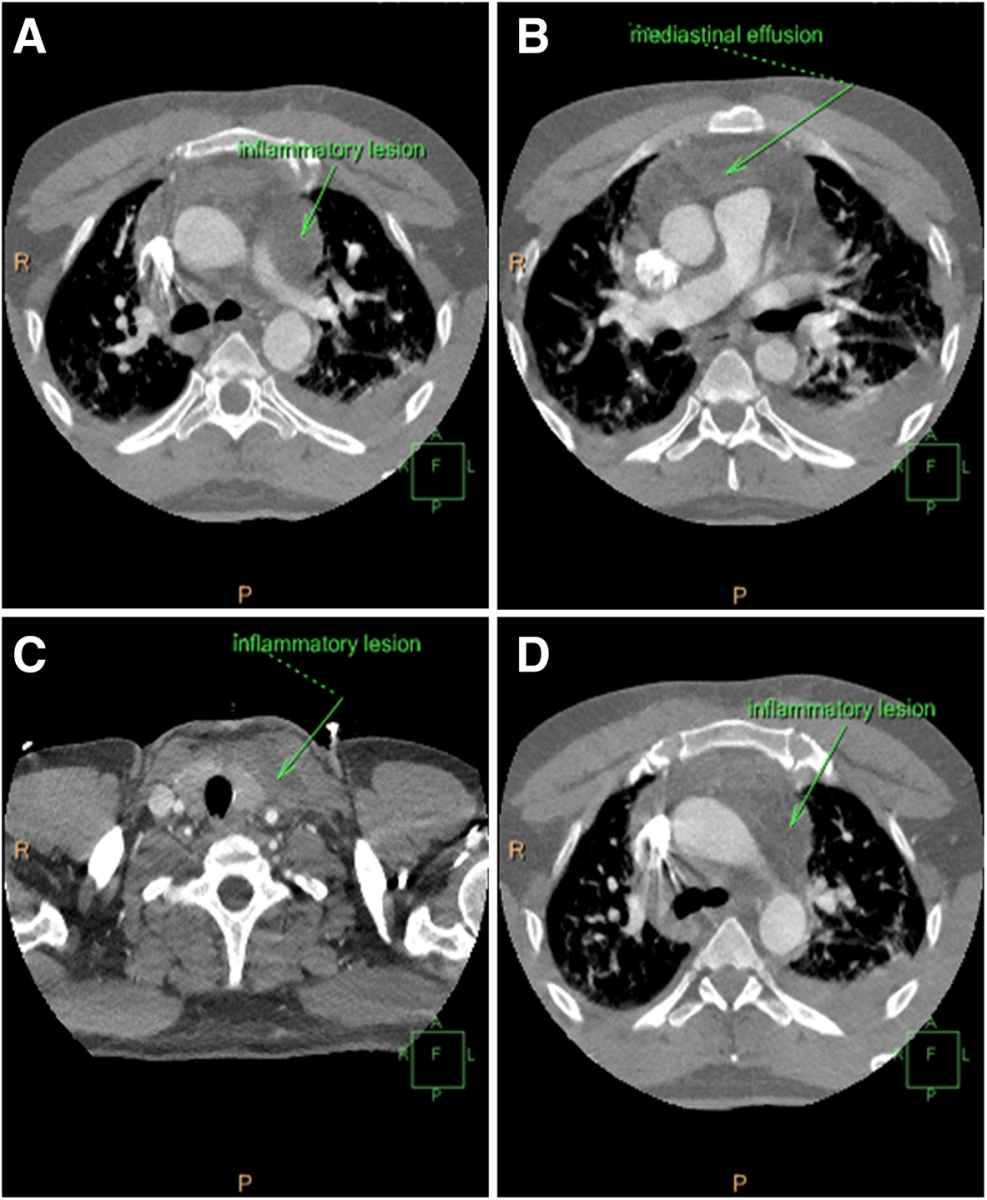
Preoperative computed tomographic (CT) scans of a critically ill patient displaying clinical signs of descending necrotizing mediastinitis. a CT scan showing increased density of the middle mediastinal space. Fluid collections in mediastinum and lymphadenopathy are shown (*arrow*). b CT scan showing increased density of the mediastinal space, fluid collections, and lymphadenopathy with bilateral pleural effusions (*arrow*). c CT scan showing fluid collections, as well as abscess formation left of the thyroid gland and ventral of the carotid arteries (*arrow*). d CT scan showing increased density of the middle mediastinal space, fluid collections in mediastinum, and lymphadenopathy (*arrow*)
